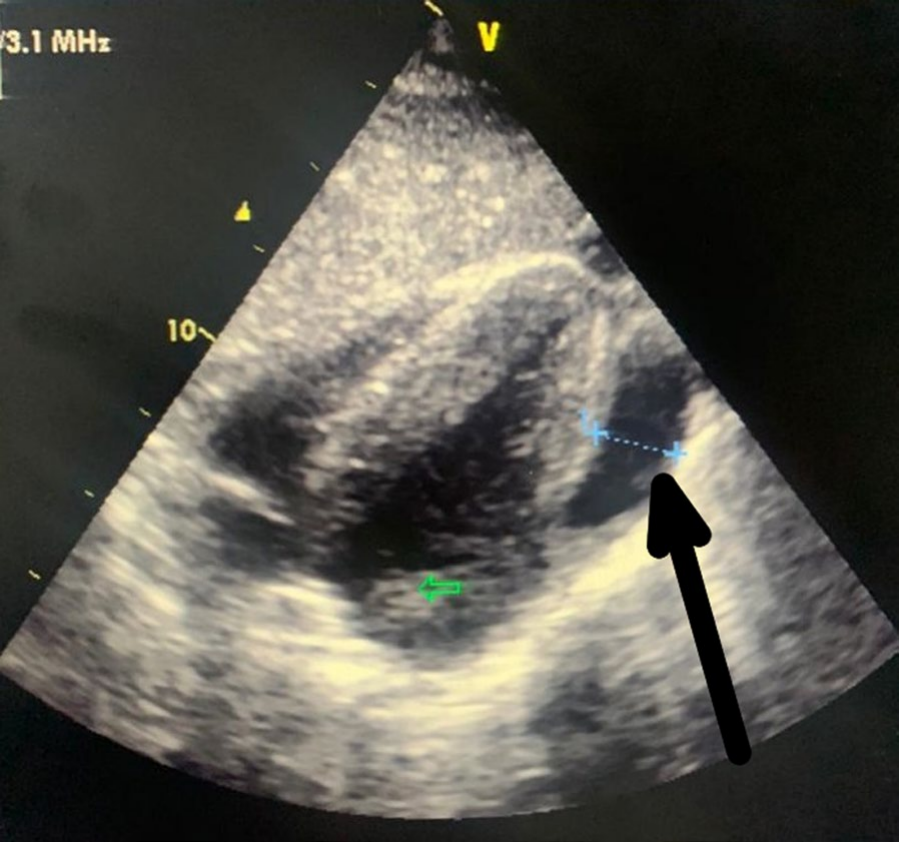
Transthoracic echocardiography showing the pseudoaneurysm of the left ventricular free wall (arrow)
